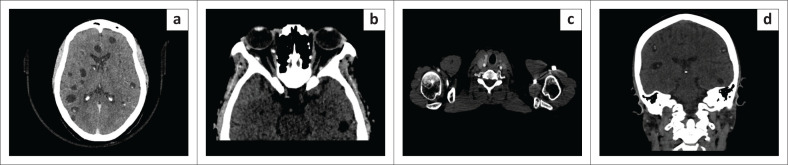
Axial CT brain (a) with the classic appearance of thin-walled cystic lesions with central hyperdensity representing a scolex, as well as focal round calcifications. Other sites of the head and neck manifestations of the disease are demonstrated with further classic appearing thin-walled cystic lesions with central hyperdensity representing a scolex on axial CT brain (b) within the retroocular structures (medial and lateral oblique muscles), axial neck CT (c) within the thyroid gland and on the coronal image (d) within both parotid glands.
